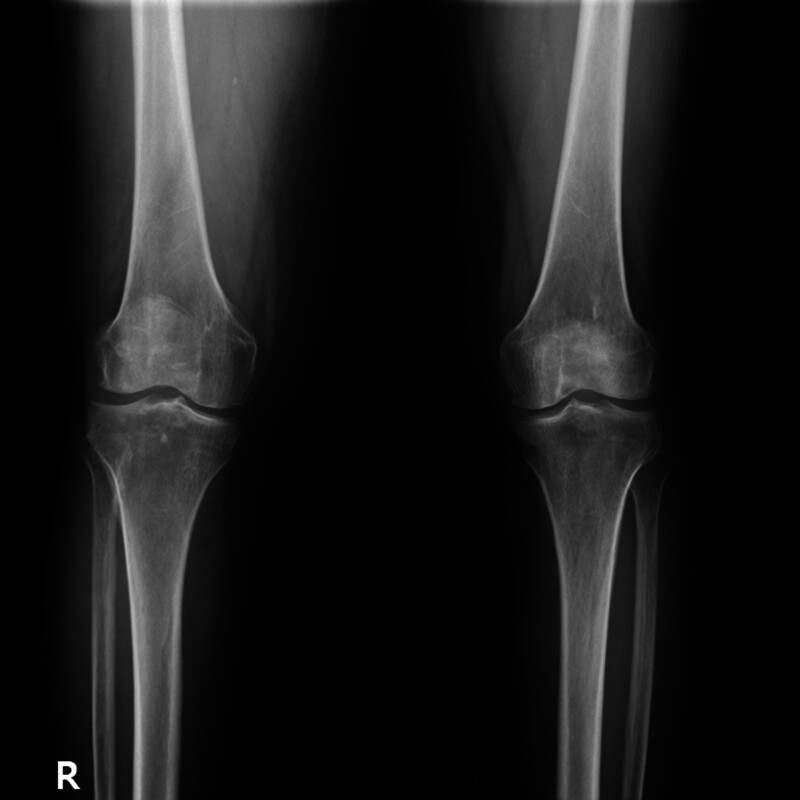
Knee X-ray in AP view, showing bilateral osteoarthritis (Kellgren–Lawrence grade II).
